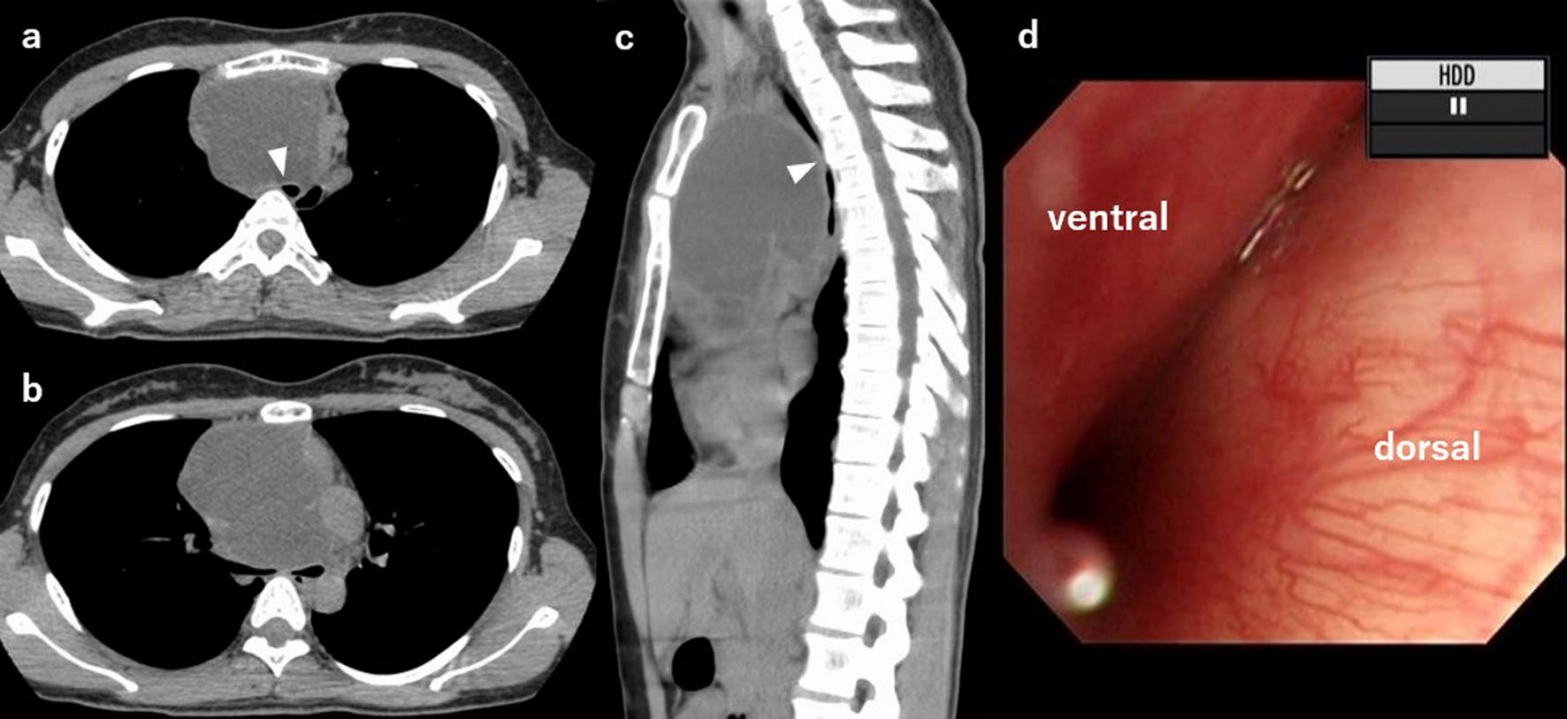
CT and bronchoscopy findings before drainage. a CT image at the trachea level. A giant anterior mediastinal cystic mass compressing the trachea was noted. The trachea was deviated to the left (white arrowhead). b CT image at the level of carina of the trachea. c Sagittal section of the CT image. The trachea was compressed (white arrowhead). d Bronchoscopy image at the trachea level. The trachea was compressed and it was difficult to observe the right main bronchial inlet. *CT* computed tomography
